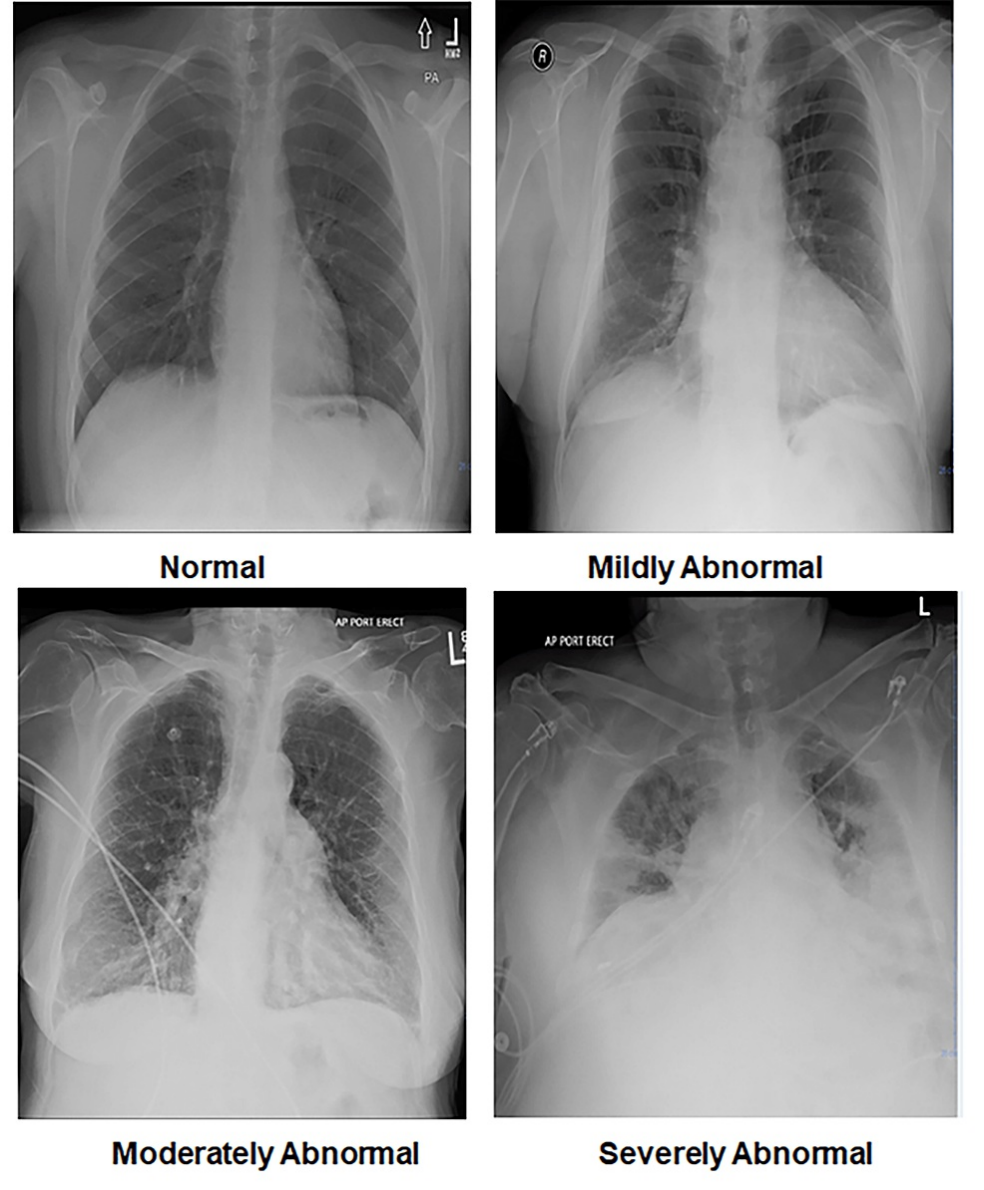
Examples of normal, mildly abnormal, moderately abnormal, and severely abnormal chest X-ray images from influenza patients. X-ray: X-radiation.
